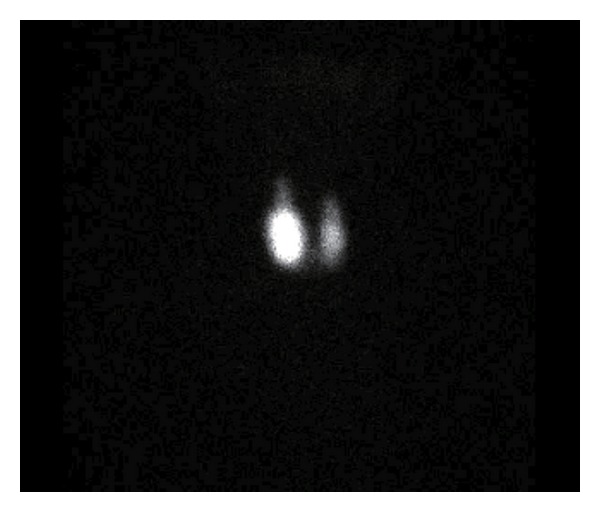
I^123^ scintigraphy of the thyroid gland. There was a strong uptake corresponding to the tumor in the right lobe detected on the ultrasonography. A nonsuppressible uptake was also observed throughout the remaining thyroid gland, thus suggesting underlying Graves' disease. The total uptake percentage of I^123^ for four hours was approximately 24% (range: 4–16%).
